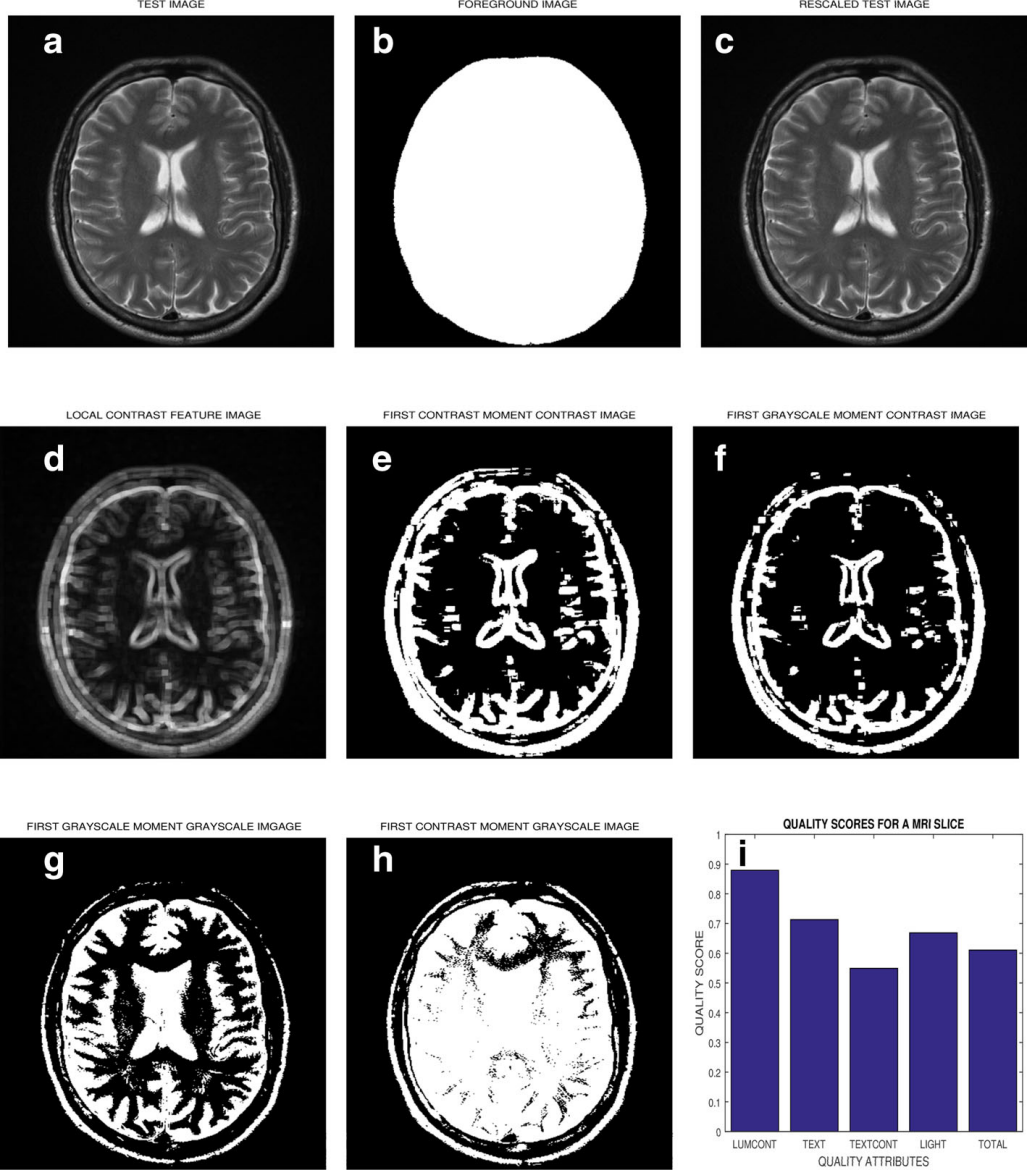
The different stages of the algorithm for post-acquisition quality evaluation of a brain MRI slice. a The test image has its (b) foreground extracted. c The test image in (a) has its pixel intensity levels rescaled to lie between 0 and 1. d Grayscale and contrast feature images are extracted from the test image. Duplicating the rescaled image in (c) extracts the grayscale image at no computational cost. e, f, g and h are the four binary feature images generated by using the first moments of the feature images in (c) and (d) as global thresholds. i Luminance contrast, texture, texture contrast, lightness and total quality scores are computed by matching relevant pairs of the feature images in (e), (f), (g) and (h)
